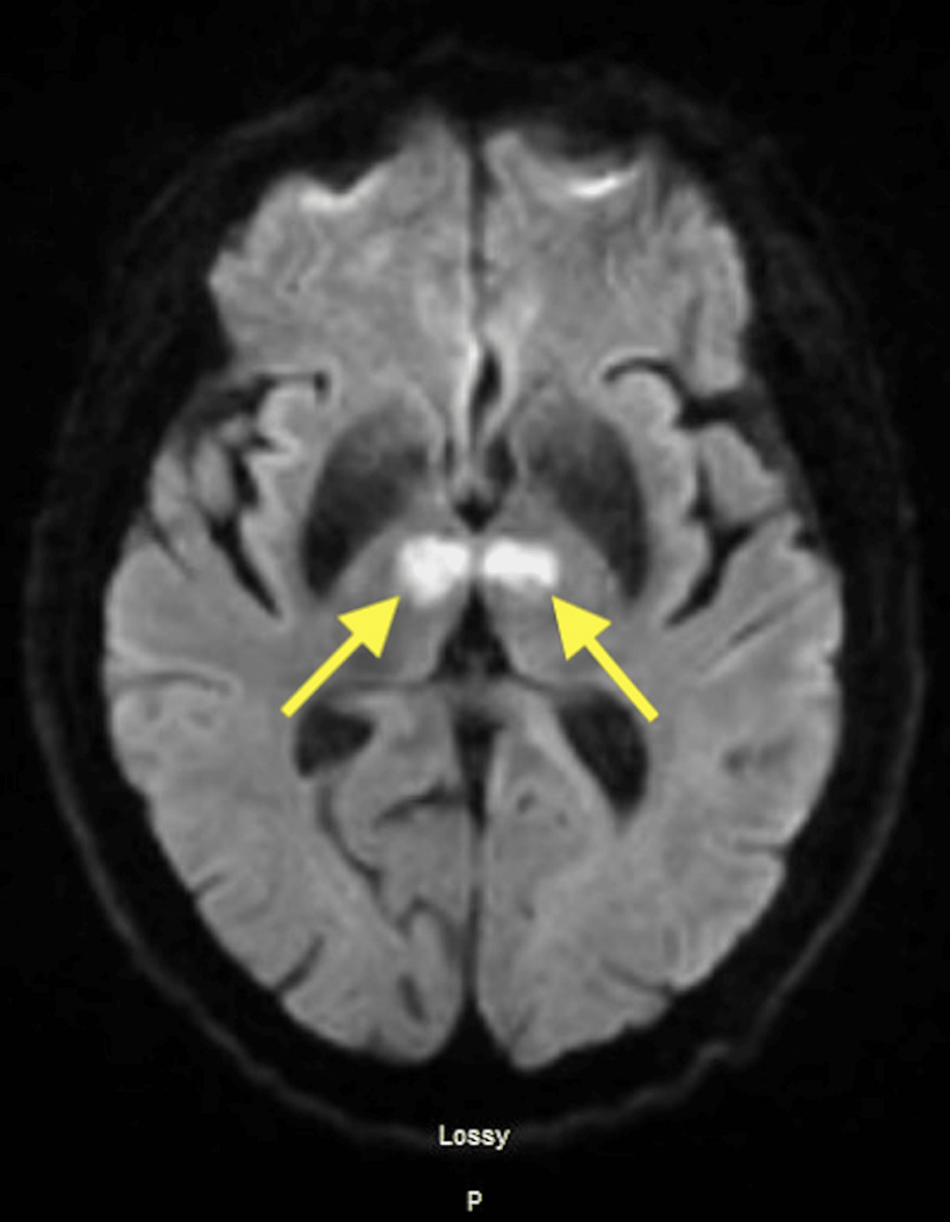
MRI of the brain without contrast demonstrating an area of restricted diffusion on DWI sequence within bilateral thalami reflecting artery of Percheron infarct Restricted area of diffusion seen on DWI sequence (arrows) MRI: magnetic resonance imaging. DWI: diffusion-weighted imaging
